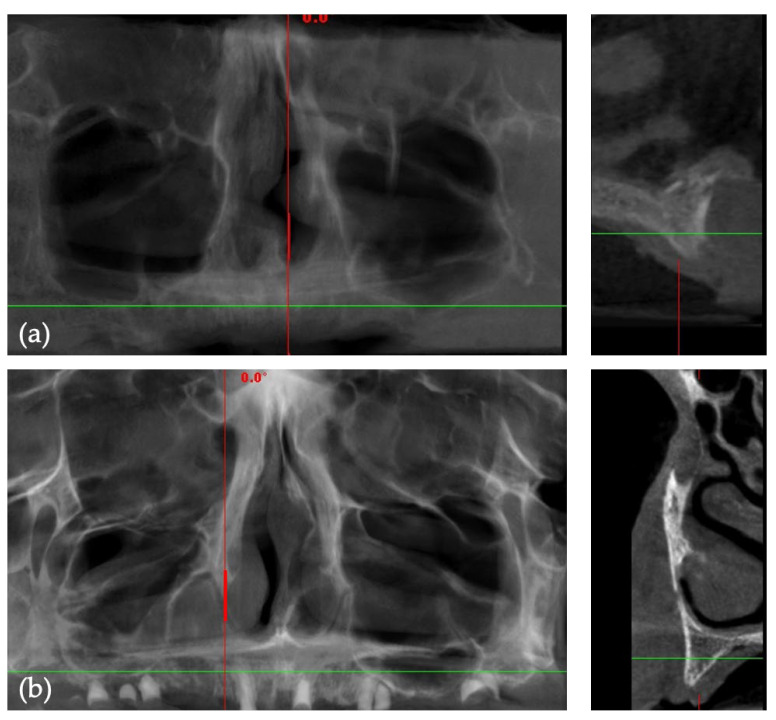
Pre-surgical CBCT images: (a,b) panoramic (green lines) and cross-sectional (red lines) views.
